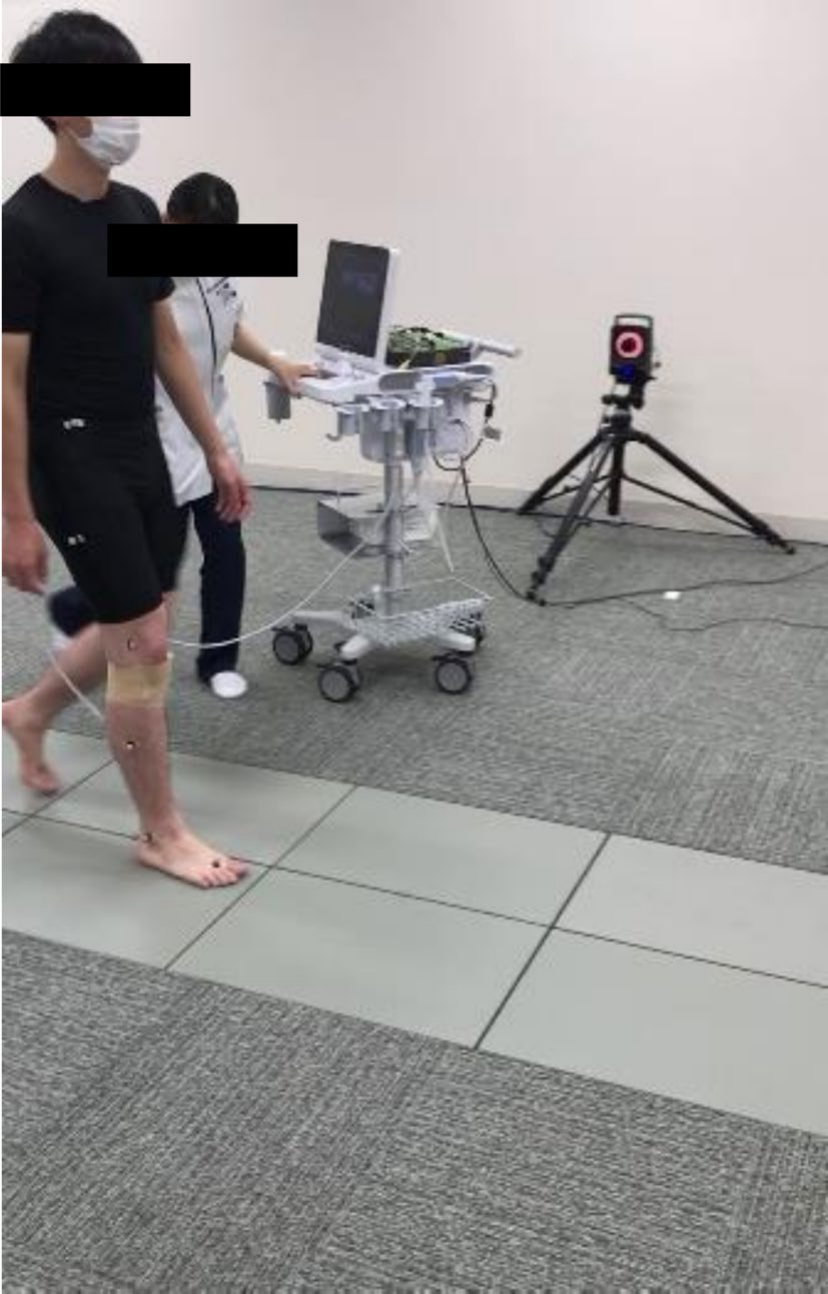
Dynamic ultrasonographic imaging. The measurement image of dynamic evaluation of meniscus extrusion during walking
